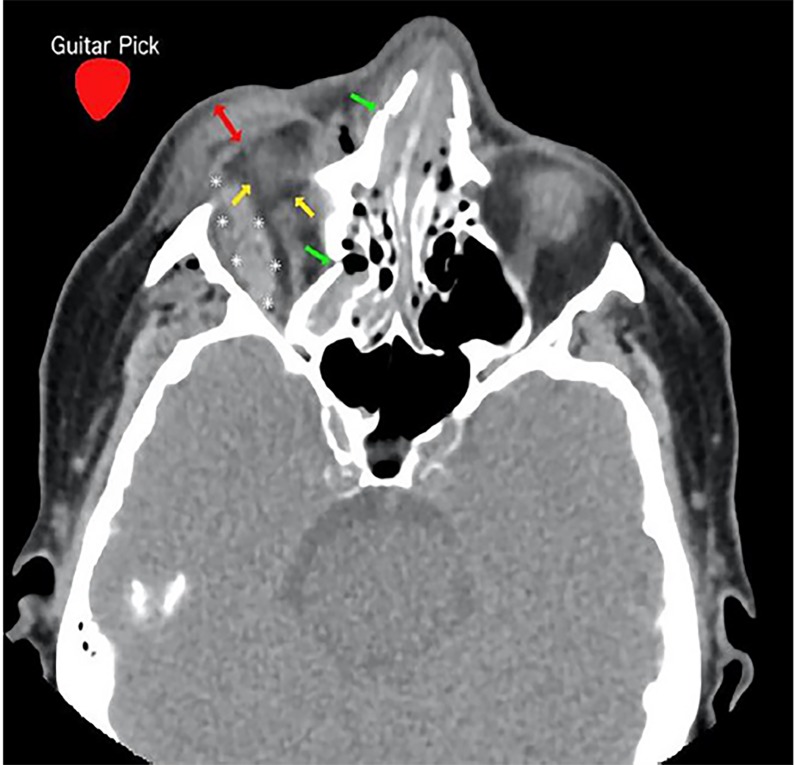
Computed tomography of facial bones without contrast, axial view, demonstrating retro-orbital hematoma (asterisks); tenting of posterior sclera (also known as “guitar pick” sign) (yellow arrows); eyelid edema (red two-way arrow); bony fractures (green arrows), and superimposed image of a guitar pick (red guitar pick with white text). The hematoma within the orbit is impinging on the orbital contents, pushing it medially and slightly superiorly.
